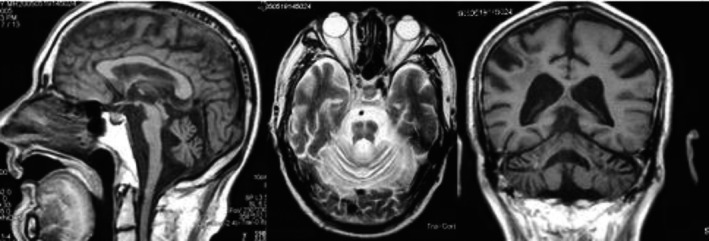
MRI images showing atrophy in the cerebellum and brainstem, “hot cross bun” sign in T_2_WI, enlargement of the fourth ventricle, and mild atrophy in supratentorial structures
